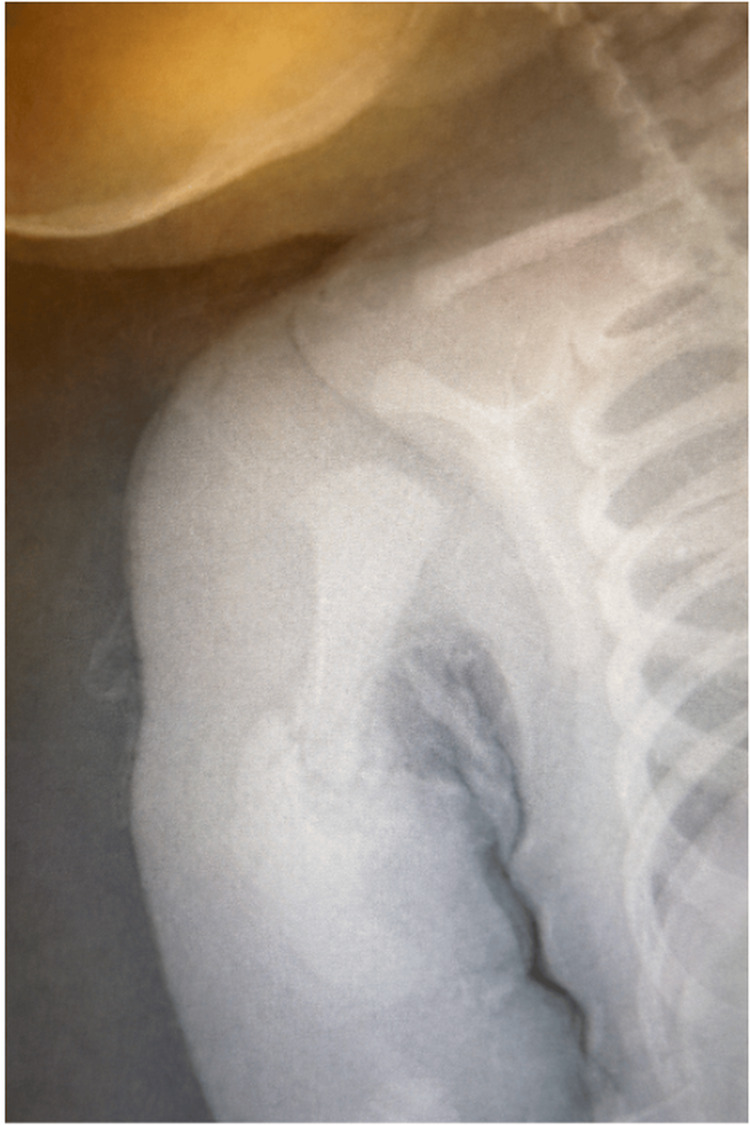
X-ray image after three weeks Healing fracture of the right humeral midshaft, with persistent angulation and proximal migration.
